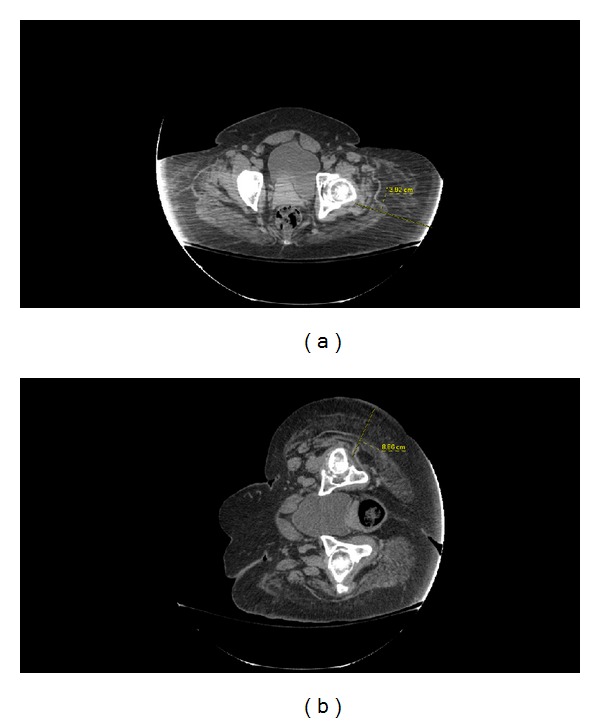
CT imaging to demonstrate soft tissue geometry and gravitational changes with position. (a) Axial supine. (b) Lateral decubitus. These scans are presented as an example and are from a black woman with BMI of 45.0 kg/m^2^.
